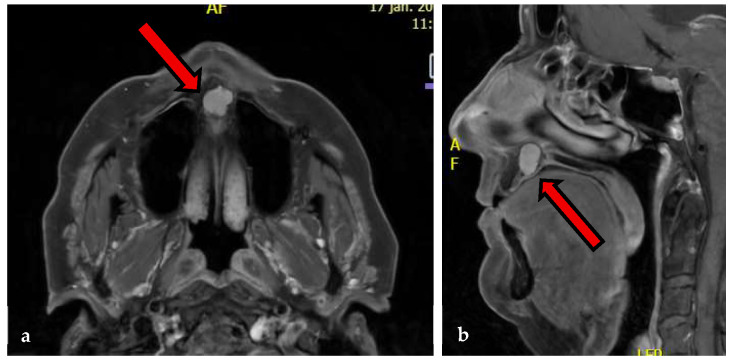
(a) Preoperative axial MRI with contrast showing an enhancing lesion (red arrow) at the anterior hard palate in close relation to the nasal septum. (b) Preoperative sagittal MRI showing lobulated contrast-enhancing lesion (red arrow) at the anterior hard palate with extension toward the nasal septum and maxillary crest.
